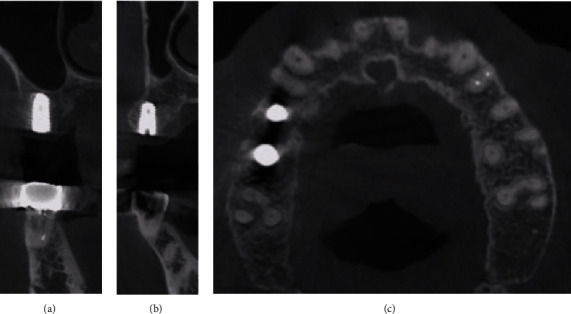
(a–c) Post-implantation radiograph views (Case 1). The width of the alveolar bone was well-maintained, and the implants were placed.
